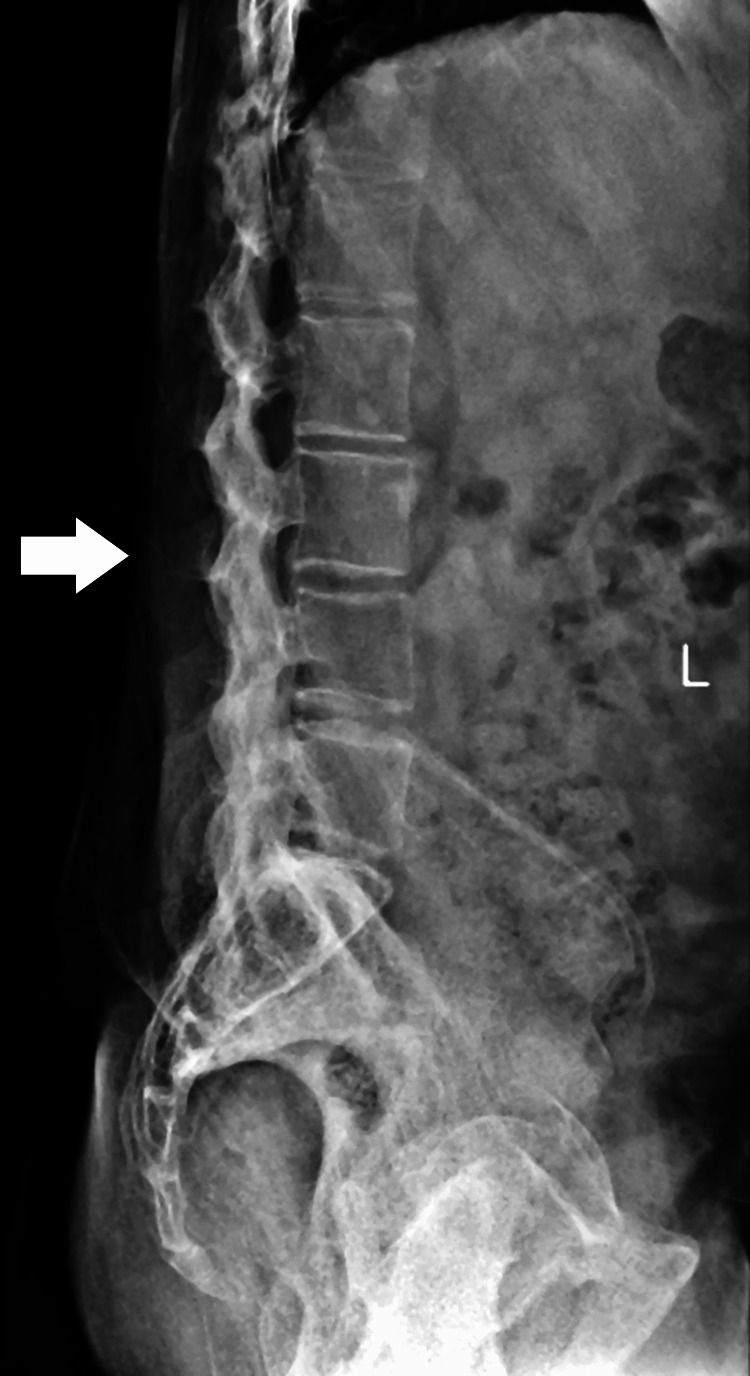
Lumbo-sacral spine X-ray AP view suggestive of ankylosed spine AP: anteroposterior
